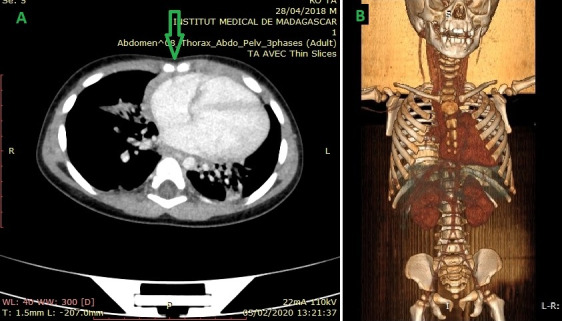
Chest axial computed tomography (CT) scan (A) demonstrates lack of fusion of the sternal
